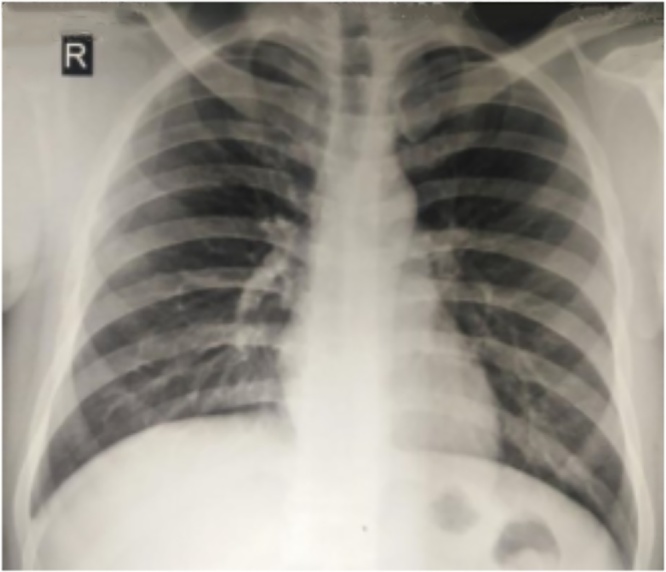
Chest radiograph showed no lung metastases.
